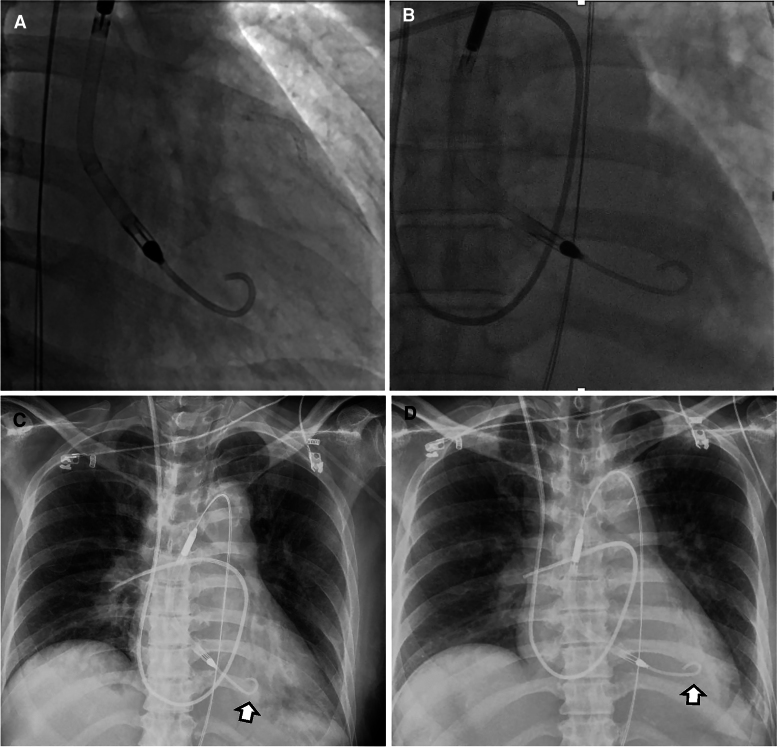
Fluoroscopic and radiographic imaging of Impella CP positioning. (*A*, *B*) Fluoroscopic images during initial placement (*A*) and after repositioning (*B*). (*C*, *D*) Chest radiographs showing Impella CP tip (arrows) before (*C*) and after (*D*) repositioning. The device was initially placed in a shallow position due to arrhythmias suspected to result from myocardial wall contact. Repositioning advanced the catheter slightly, orienting the tip towards the posterior wall.
